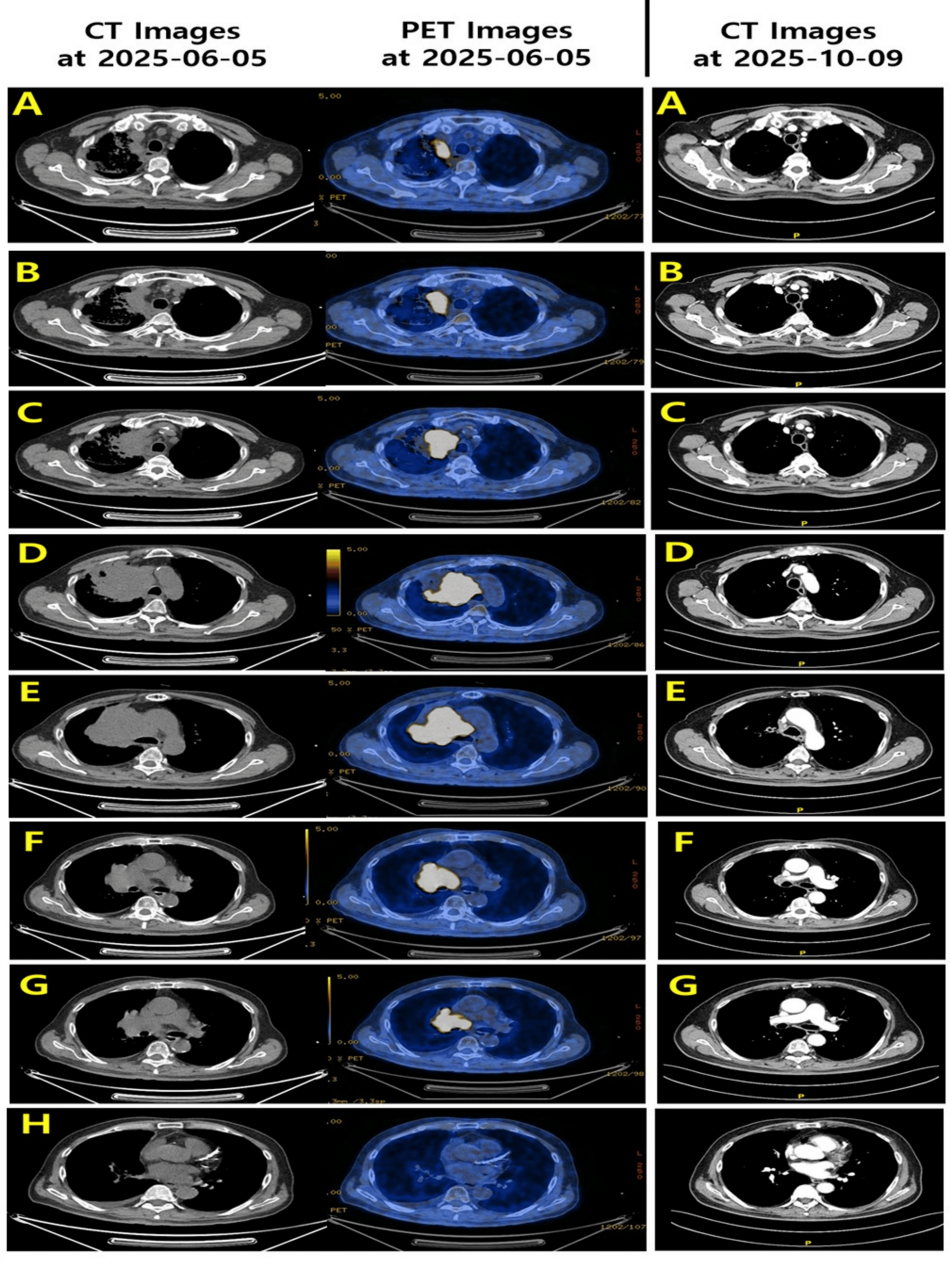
Serial axial CT and PET/CT images comparison before and after treatment (slices A–H) Panels A–H represent matched axial levels from the thoracic inlet to the cardiac upper border. The left two columns show baseline images obtained on June 5, 2025, including (1) contrast-enhanced CT and (2) corresponding positron emission tomography (PET/CT) fusion images. These baseline scans reveal a large, irregular anterior mediastinal mass with heterogeneous soft-tissue density and intense radiotracer uptake, consistent with aggressive metabolic activity of extensive-stage small-cell lung cancer. The right column shows follow-up CT images obtained on October 9, 2025, after five cycles of platinum–etoposide–atezolizumab therapy followed by consolidative radiotherapy. Across all slice levels (A–H), the previously identified tumor has nearly completely regressed, with restoration of normal mediastinal contours, resolution of paratracheal mass effect, and no residual measurable soft-tissue lesion. Lung aeration is markedly improved, and no new intrathoracic lesions are identified. This figure demonstrates a near-complete radiologic response both anatomically and metabolically, highlighting the patient’s exceptional treatment response.
